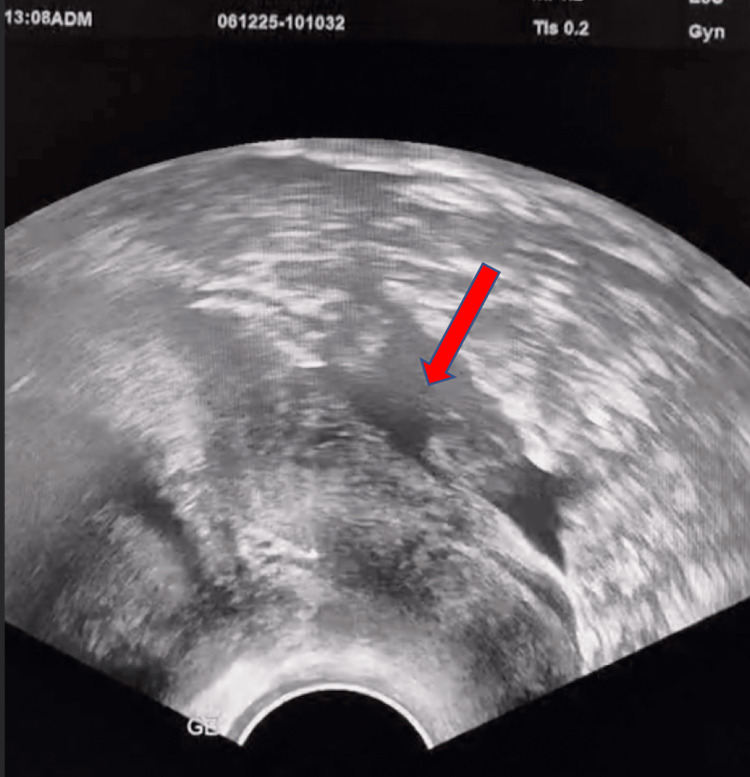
Transvaginal ultrasound revealed a moderate pelvic effusion (red arrow).
